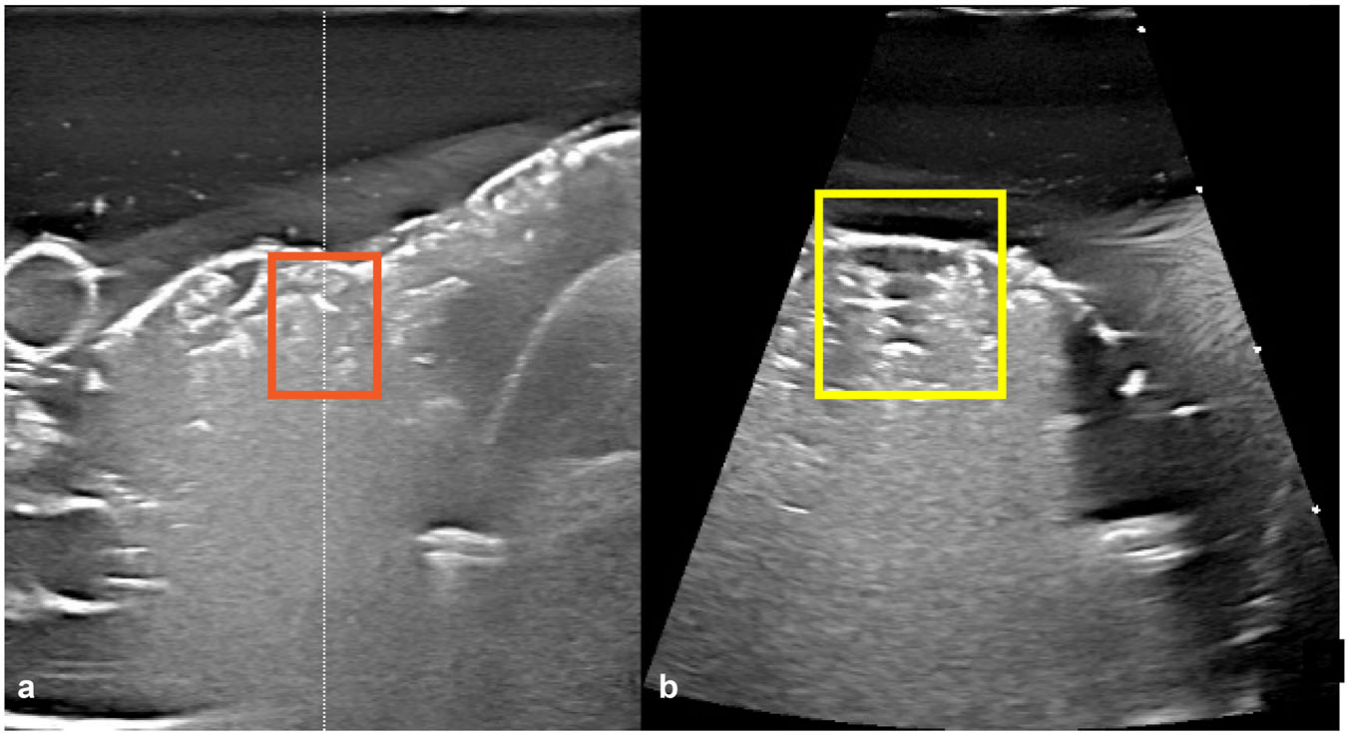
An example of the dual imaging plane potential (three-dimensional ultrasound), where on the standard transversal plane (a), the area surrounding the white dashed line (orange box) appears to be fully ablated. However, the sagittal plane (b) shows a small hypodense area (yellow square) that has not been ablated
